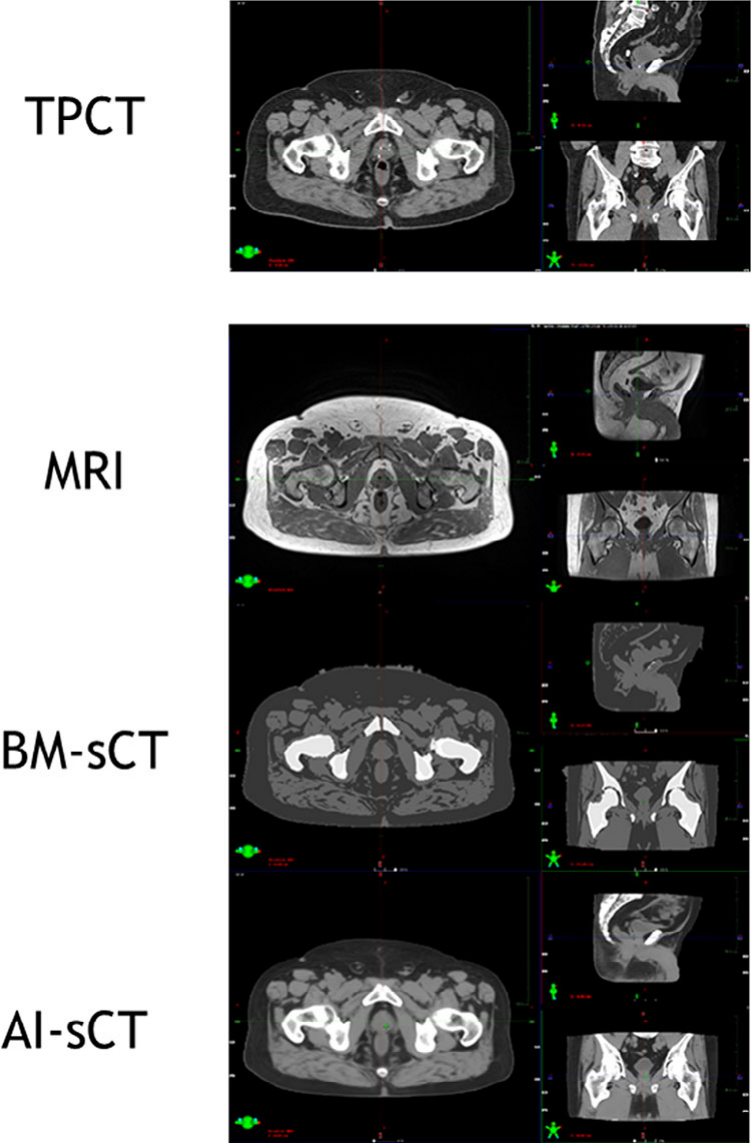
Example case of sCT algorithms on pelvis area. From the top images of treatment planning CT, MRI, BM‐ sCT, and AI‐ sCT. AI, artificial intelligence; BM, bulk‐density‐method; CT, computed tomography; MRI, magnetic resonance; sCT, synthetic computed tomography.
